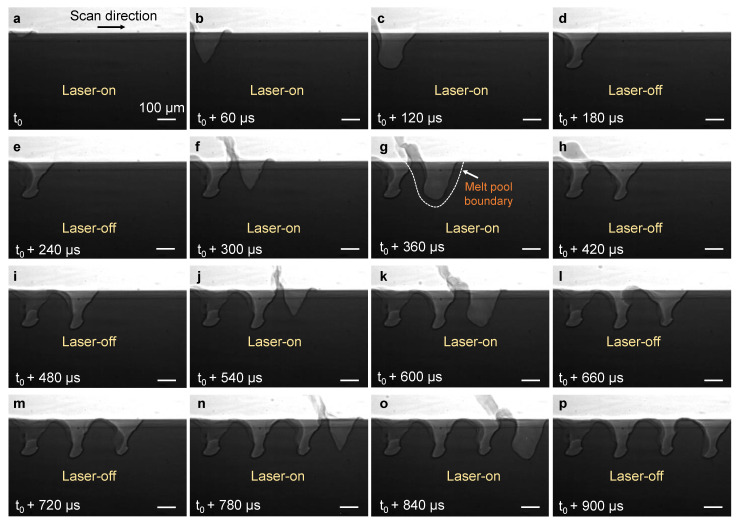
Cavity pattern formation at low laser frequency: (a–p) Dynamic X-ray images showing formation of cavity during pulsed-wave laser melting of Ti-6Al-4V substrate at a laser frequency of 4 kHz, a duty cycle of 50%, a laser power of 420 W, and a scan speed of 0.8 m/s. Note that some image frames during laser-on time and laser-off time have been skipped. The compiled movie showing the details is available in the Supplementary Material (Movie S7).
